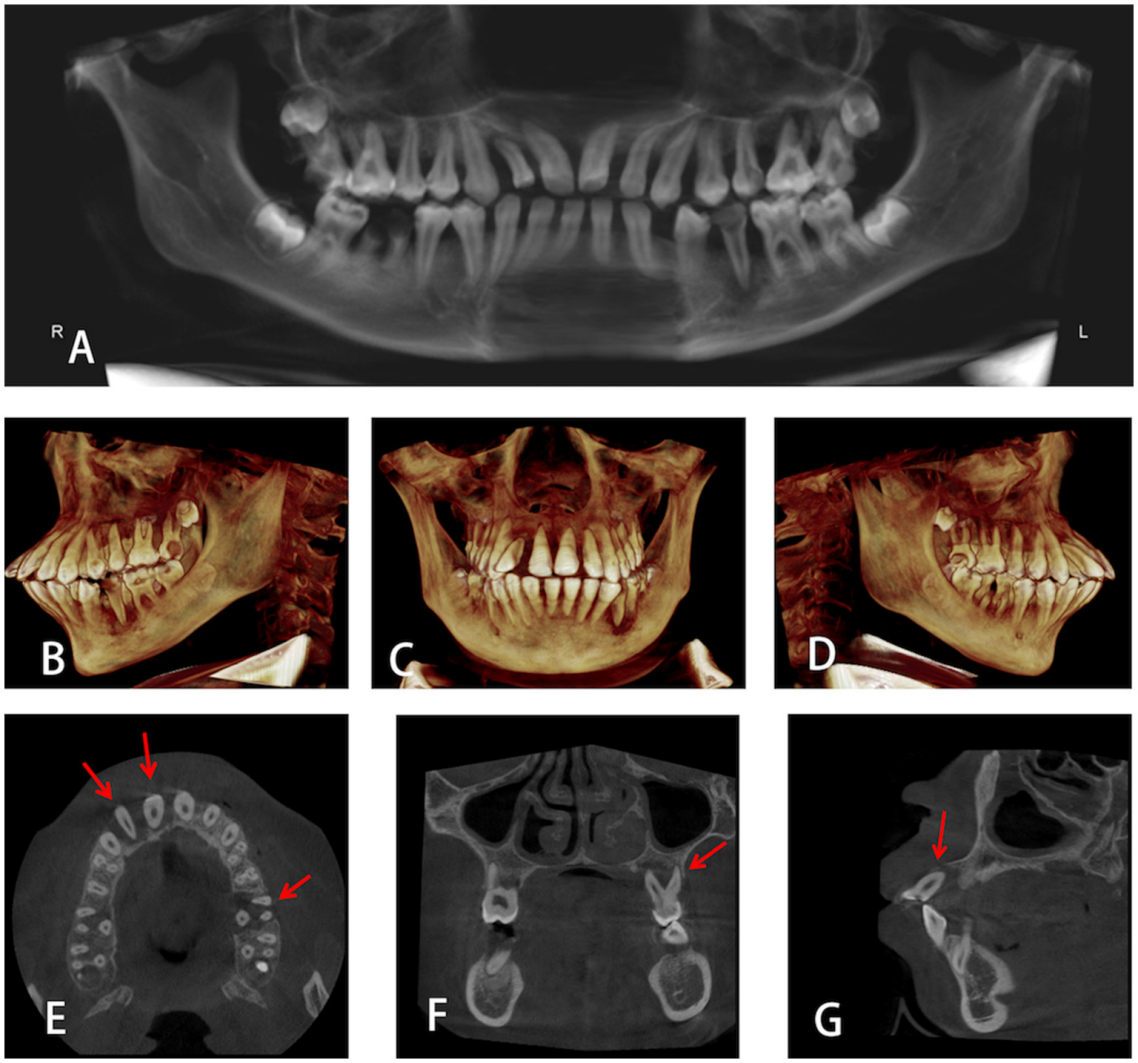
(A) Panoramic radiograph of the proband. (B–D) 3D Volumetric reconstructive cone-beam computed tomographic (CBCT) images of the whole mouth from different directions. (E–G) Alveolar bones of teeth #12, #11, and #26 were absorbed to the root apex.
